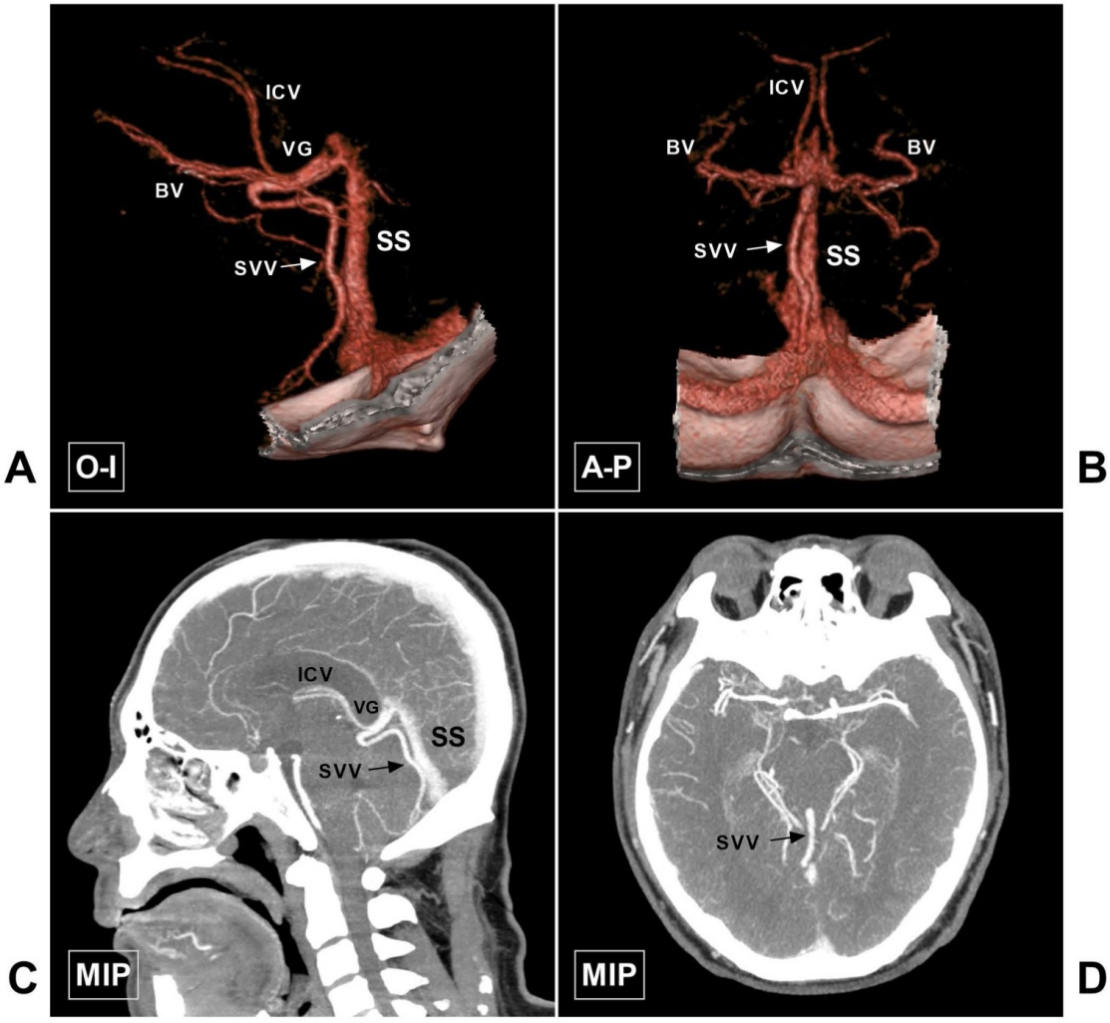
Large SVV. A-B, CTA in O-I and A-P view show a large SVV (arrow), which drains to the VG. C-D, MIP in sagittal and axial view also shows the large SVV (arrow). Abbreviations: A-P, anterior to posterior; BV, basal vein; CTA, computed tomography angiography; ICV, internal cerebral vein; MIP, maximum intensity projection; O-I, outside to inside; SS, straight sinus; SVV, superior vermin vein; VG, vein of Galen.
